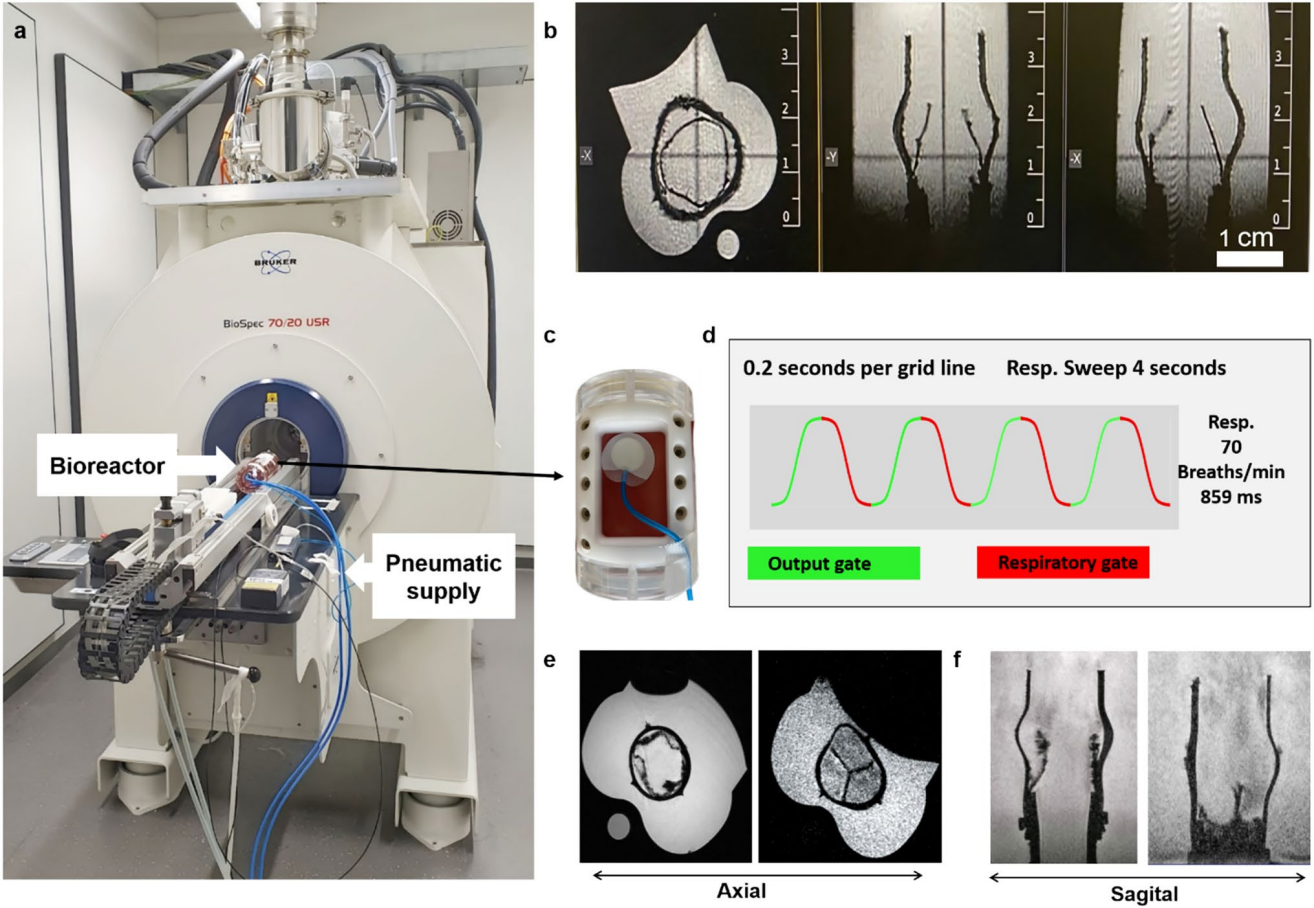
Dynamic magnetic resonance imaging. a The bioreactor going inside the 7 T MRI coil and the blue tubes are the inlet and outlet of pneumatic connections. b Localizer sequence shows the placement of the valve in MRI. c The breathing patch on the top of the ultrasound window. d The respiratory graph observation during the MRI scans. e–f Observation of the opening and closing of the valve in the MRI
